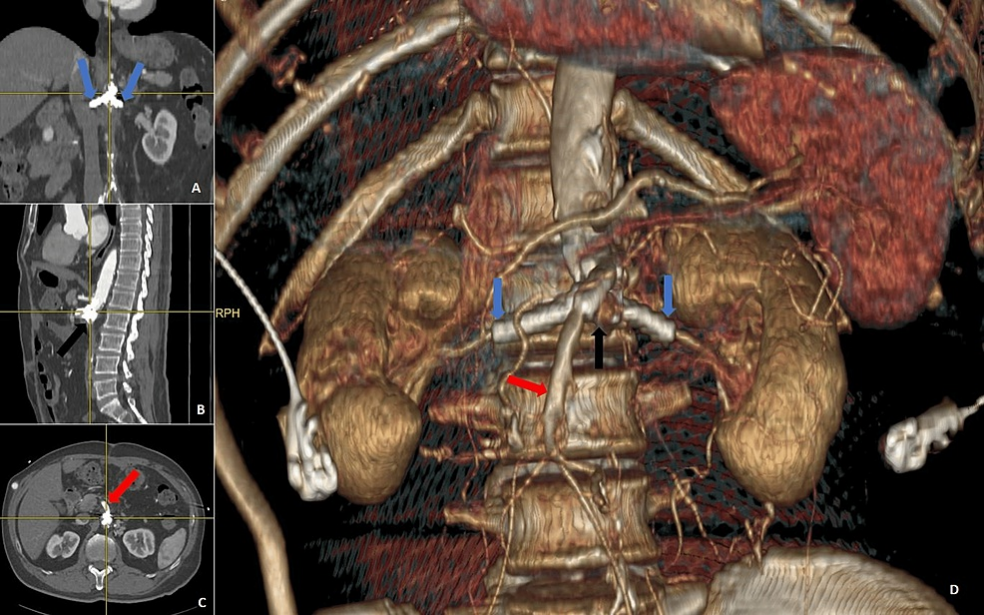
CT aortic angiogram six months post corrective surgery. (A) coronal, (B) sagittal, (C) axial, and (D) 3-dimensional reformat centered on the aortic stump (black arrow) demonstrates good perfusion of the SMA (red arrows) as well as both renal arteries (blue arrows). SMA: Superior mesenteric artery.
